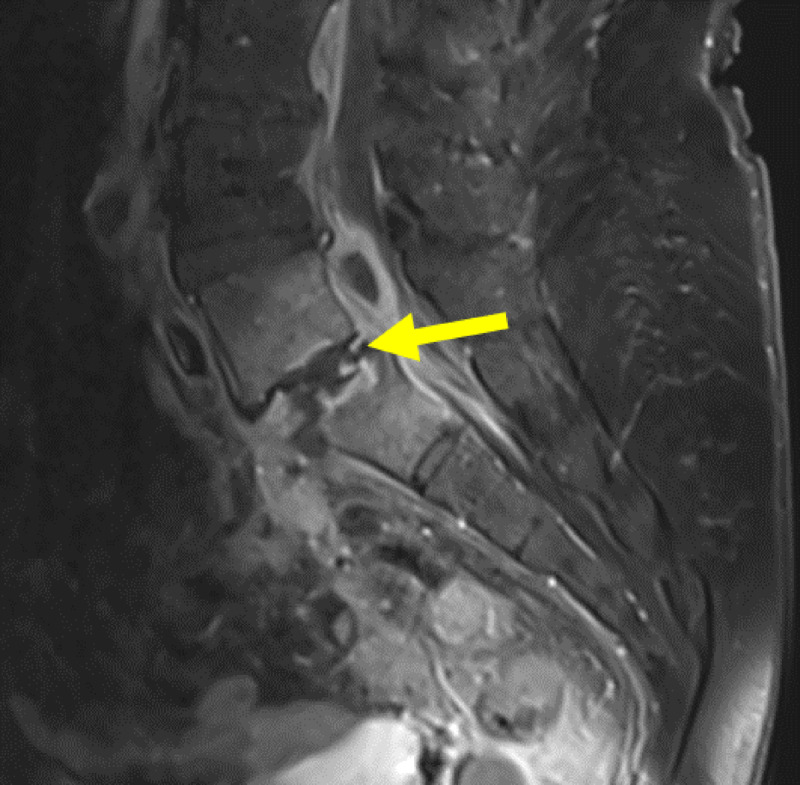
Sagittal contrast MRI in T1 fat saturation weighing: Hyperintense L5-S1 vertebrae and surrounding tissue, showing spondylodiscitis (yellow arrow). There is slight anterolisthesis of L5 upon S1.
